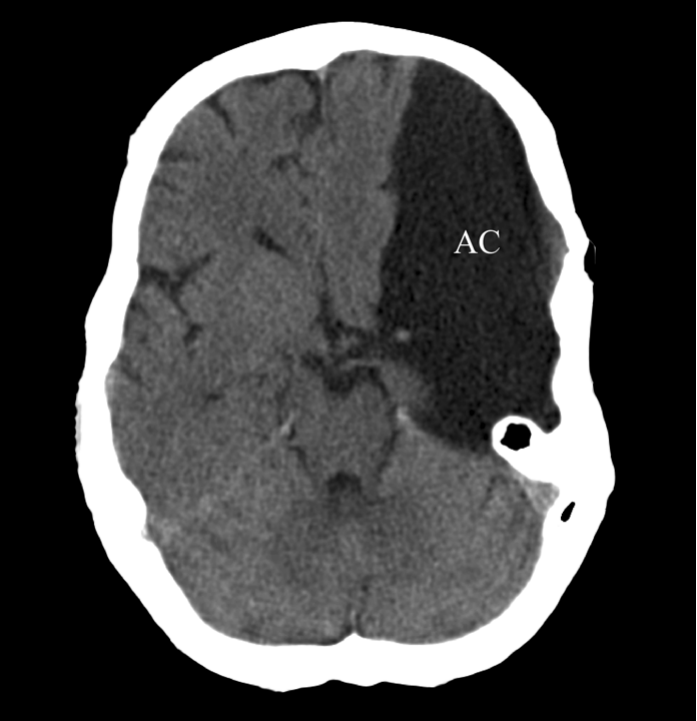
Radiological presentation of an arachnoid cyst (AC). Computed tomography (CT) scan of a large, left-sided temporal AC. Note the splitting of the Sylvian fissure and the compression of the frontal and temporal lobes, all classical features of a Galassi type 3 cyst [10]. The midline is displaced 6 mm to the right. Note the enlargement of the left cranial vault suggesting that the AC was present before the neurocranium was fully developed.
